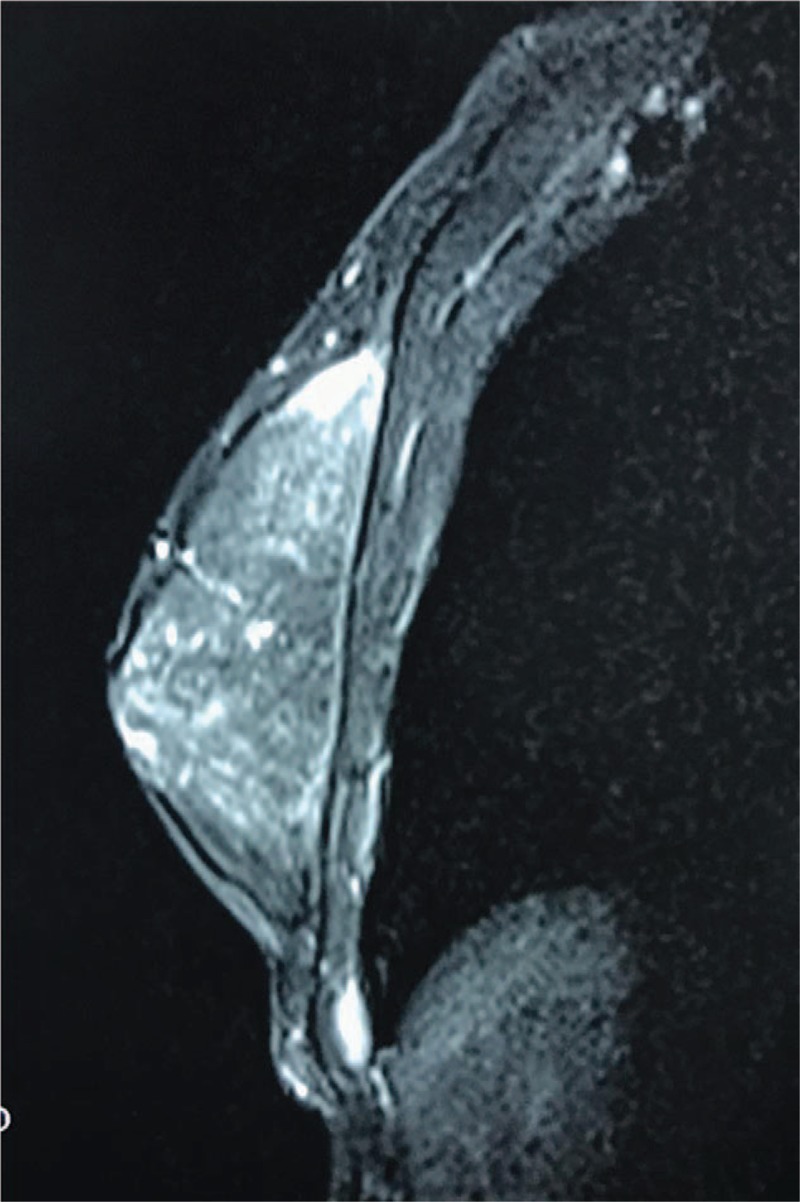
Magnetic resonance imaging (MRI) of unilateral gynecomastia (transversal plane, T1 weighted image).
